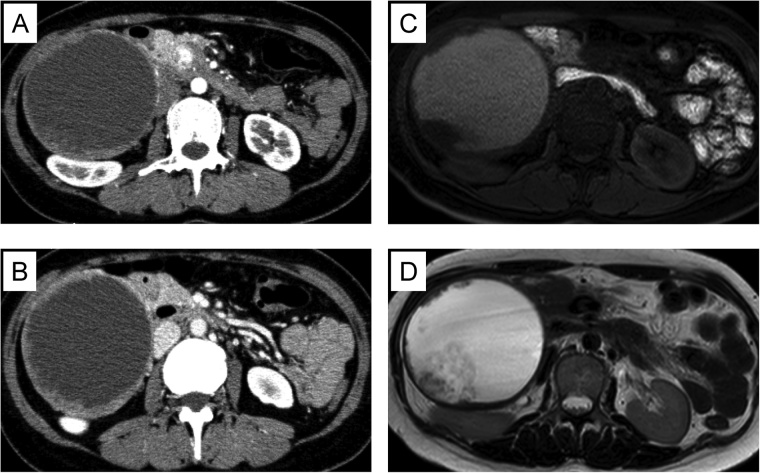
Imaging findings in CE-CT and MRI. CT showed that the huge cyst had thickened wall and many enhanced nodules. (A) Early phase. Many nodules and the thickened wall were slightly enhanced. (B) Delay phase. They were gradually enhanced. MRI showed that the intensity of intra-cystic fluid was slightly high in T1WI (C) and high in T2WI (D).
